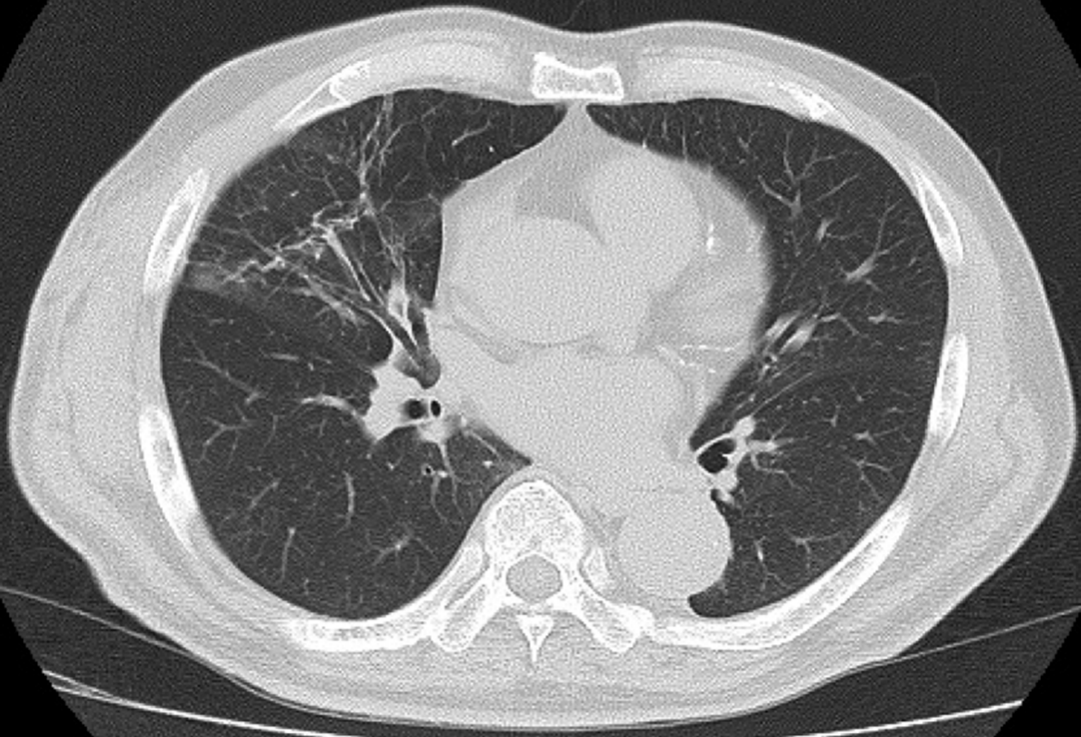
Chest computed tomography (CT) 1 year after surgical repair.
